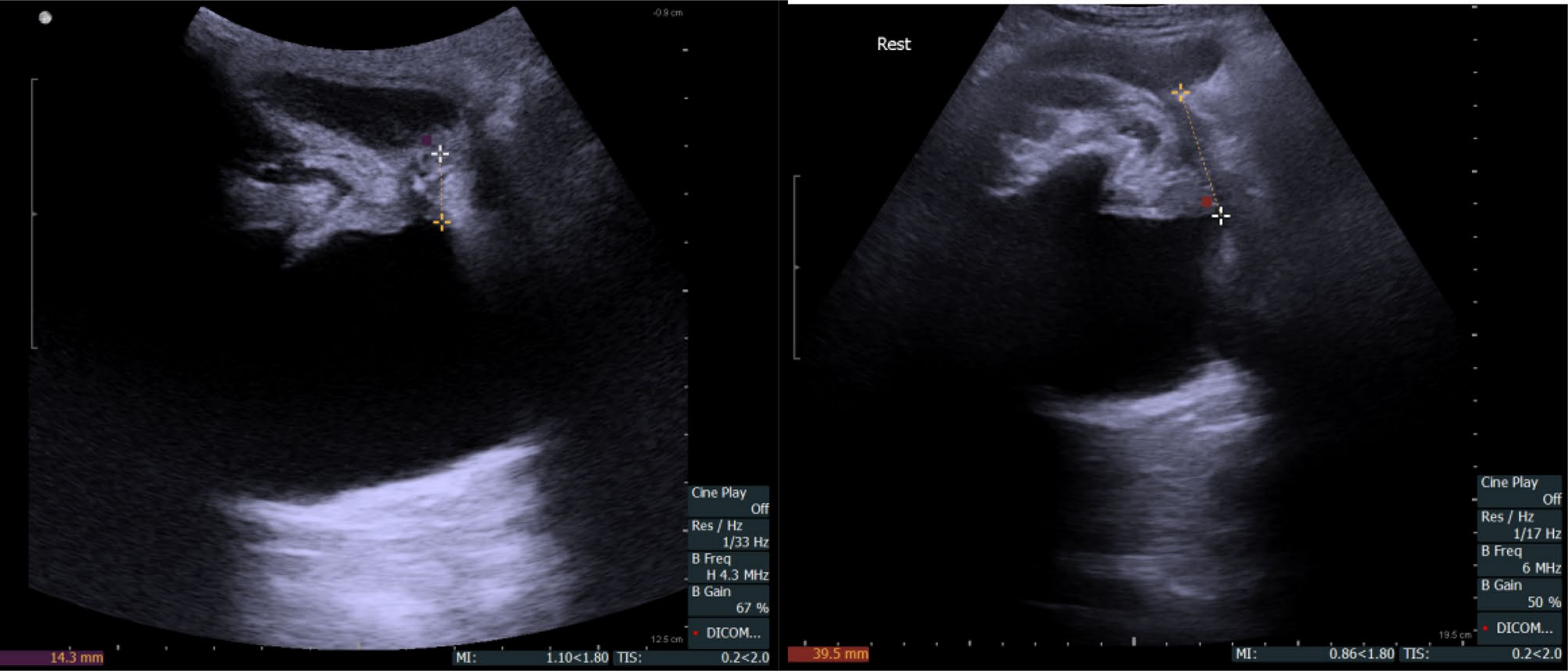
Transperineal ultrasound (TPUS) postoperative membranous urethra length (MULpost) measurement—left side, MULpost = 14.3 mm—patient incontinent at 12 months after surgery, right side, MULpost = 39.5 mm—patient continent at 1 month after surgery.
